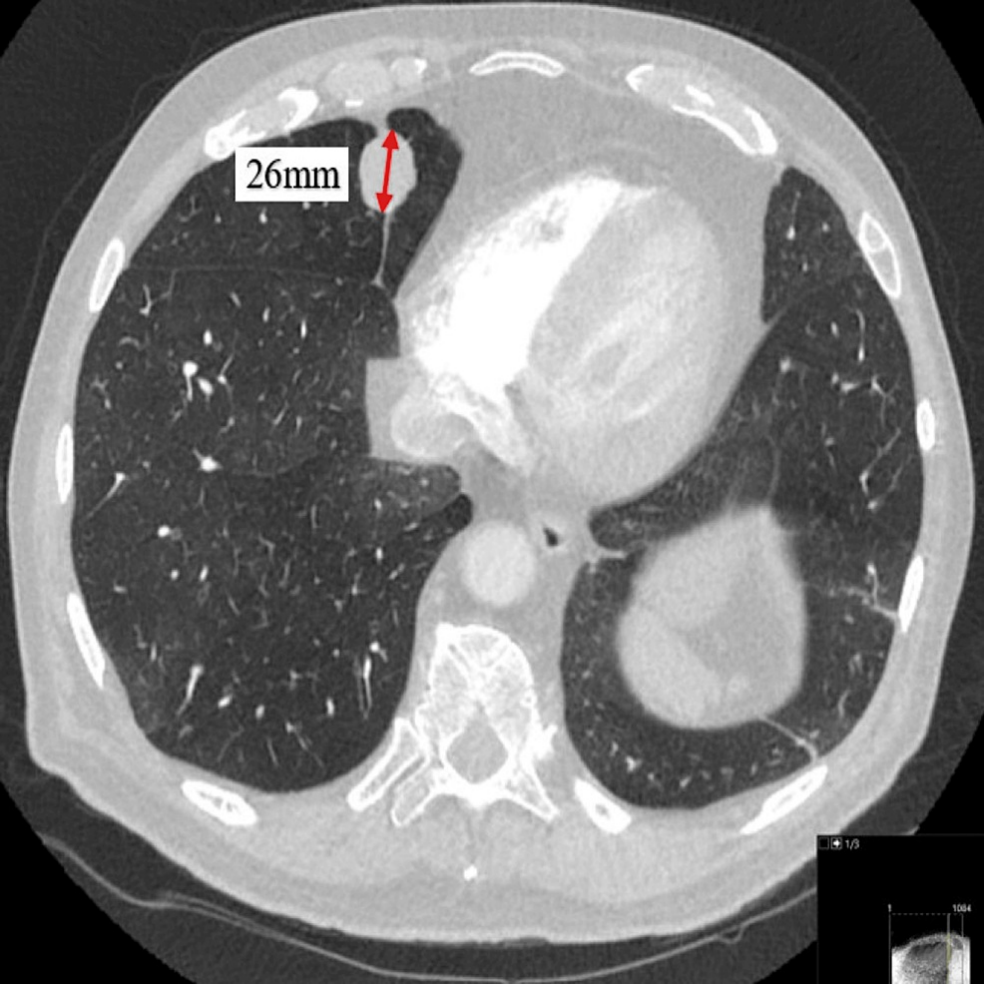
Axial computed tomogram (CT) image on lung window demonstrating an irregular well-circumscribed nodule in the medial segment of the right middle lobe measuring 26 mm (red arrow).
